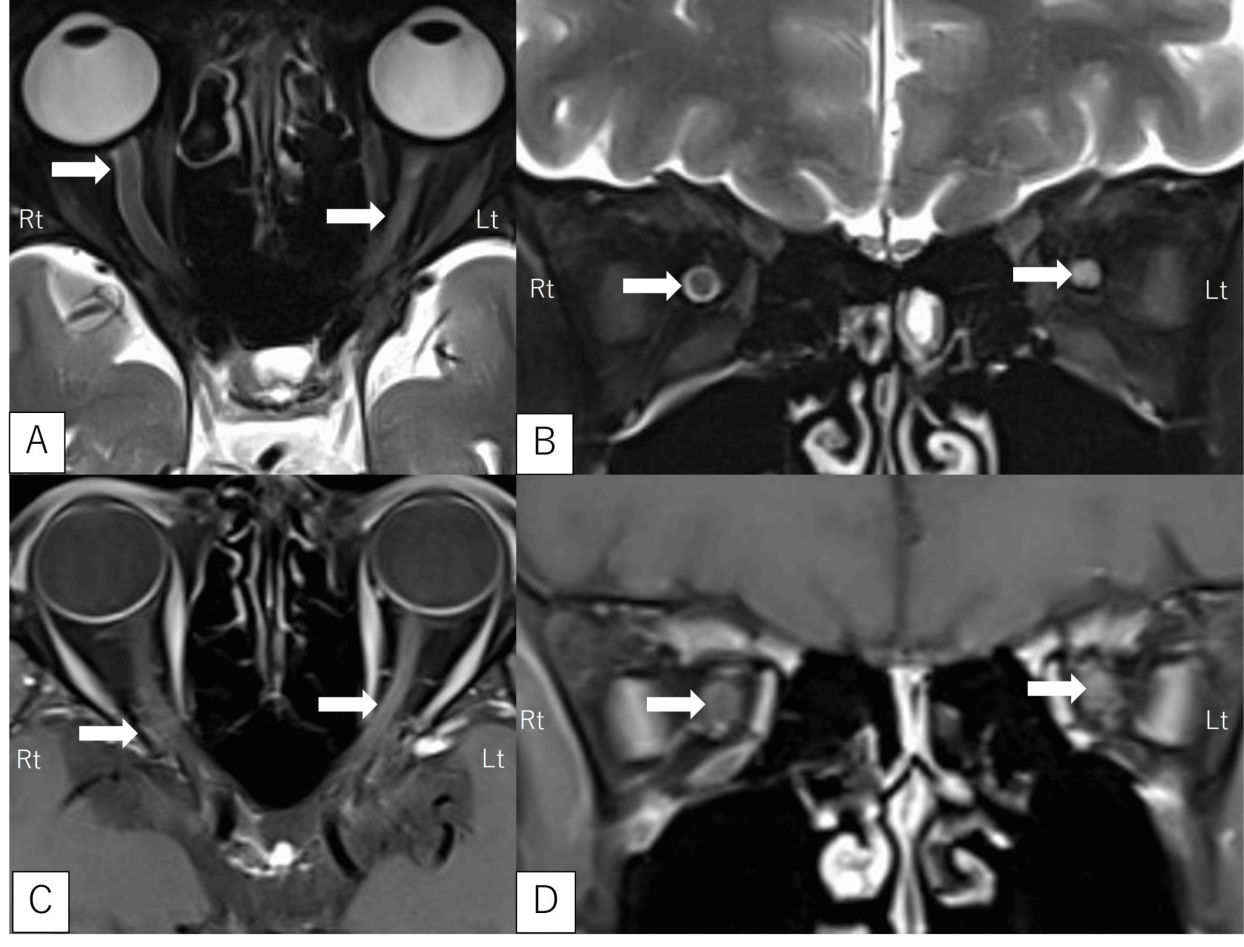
Orbital magnetic resonance imaging (MRI) findings: (A) axial and (B) coronal: short-tau inversion recovery (STIR); (C) axial and (D) coronal: gadolinium-enhanced T1WI On the right optic nerve, STIR images demonstrated high signal intensity in the perineural region of the optic nerve (A,B, arrow), and T1-weighted images with gadolinium contrast enhancement showed mild enhancement in the same area (C,D, arrow). These findings are suggestive of optic perineuritis. On the left optic nerve, STIR images revealed high signal intensity within the optic nerve itself, and T1-weighted images with gadolinium contrast demonstrated mild enhancement in the corresponding area (arrow), suspected of optic neuritis. MRI showed a suspicious finding of optic perineuritis in the right eye and optic neuritis in the left eye; however, they did not respond to immunotherapy, and a diagnosis of Leber hereditary optic neuropathy was made based on genetic testing. T1WI: T1-weighted imaging
